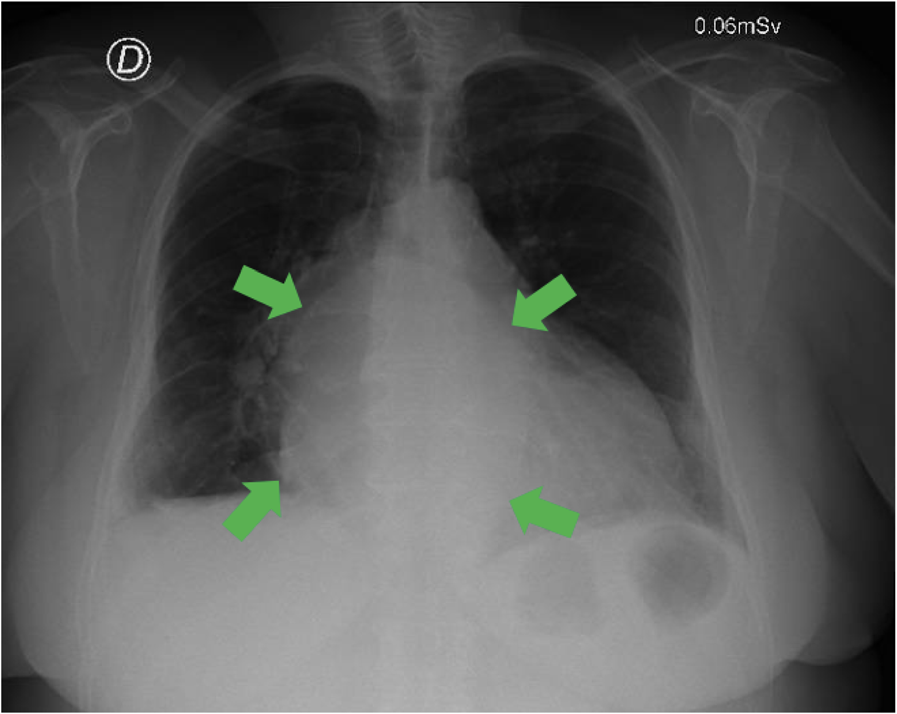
Chest X-Ray. Retrocardiac opacity indicated by green arrows, suggestive of a giant mass
